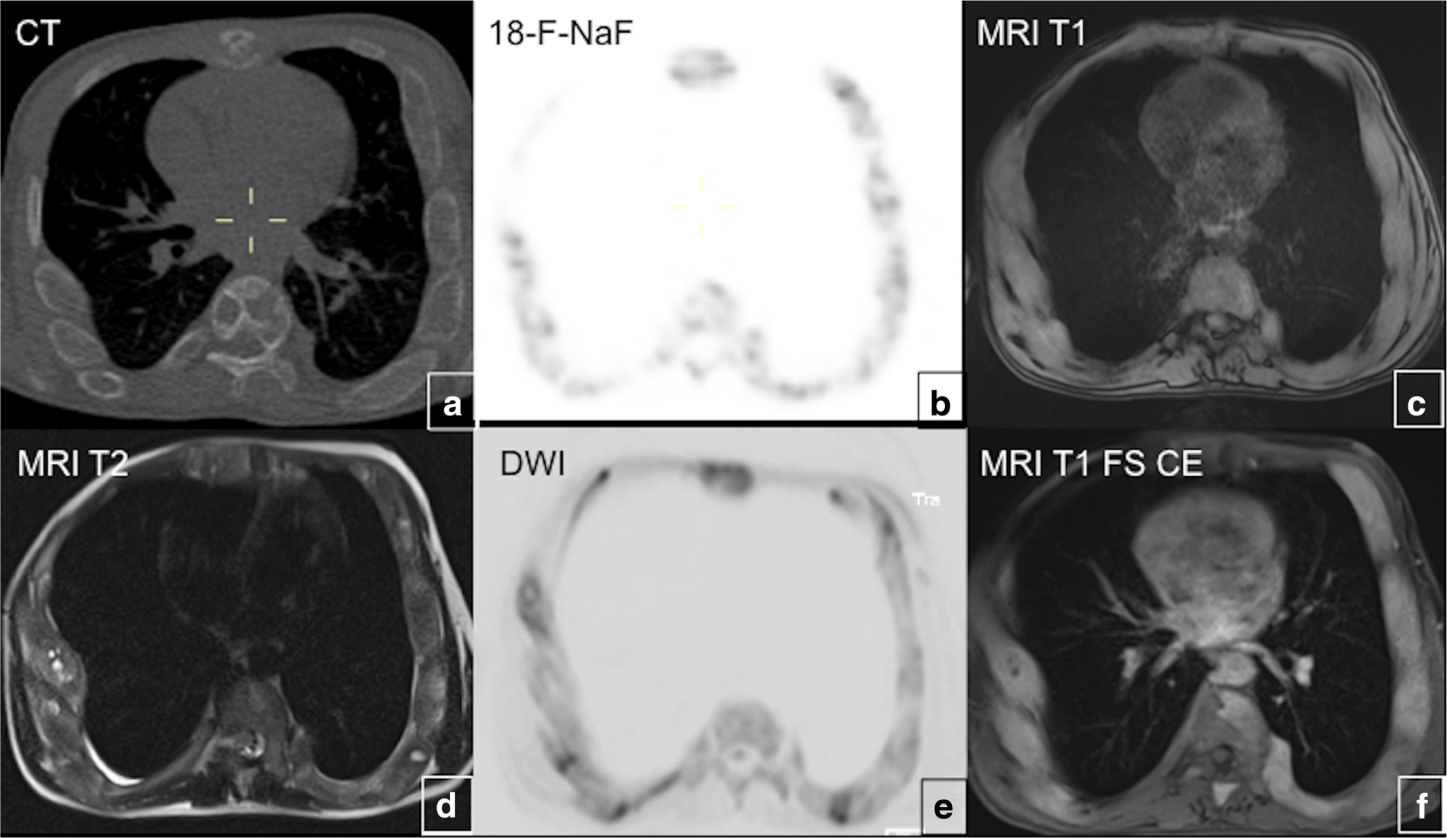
Multimodality imaging in polyostotic fibrous dysplasia (FD). a, b PET/CT with 18-F-NaF demonstrates multiple lucent FD lesions seen on CT with corresponding areas of mild radiotracer uptake on PET. c–f Lesions demonstrate intermediate T1 signal intensity on MRI (c), intermediate-to-low signal intensity on T2 (d), slightly hyperintense signal intensity on DWI (e), uniform enhancement after contrast administration (f)
